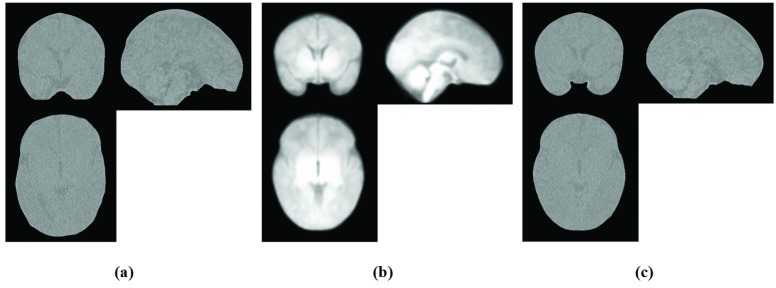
CT-MR intracranial co-registration, first step: Intracranial intensity-based registration. (a) The input moving CT intracranial space. (b) The reference MR intracranial space. (c) The CT intracranial registered to GRAMFC_T_39-42_ intracranial space using affine and non-linear SyN approach [54].
